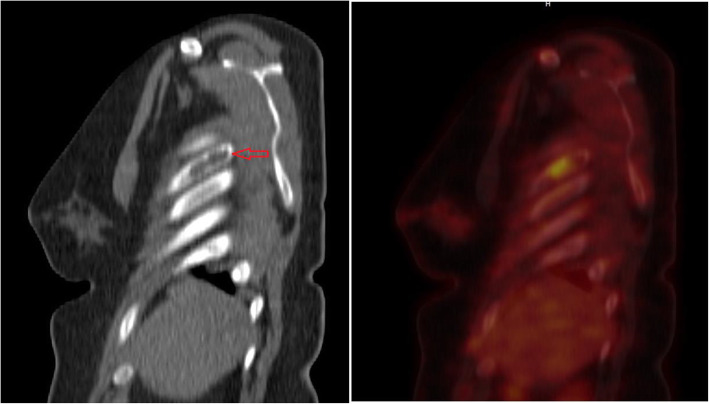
Sagittal computed tomography image and sagittal fused ^18^F-FDG PET/CT image: There is FDG-avid lytic lesion in lateral curvature of the left fourth rib
